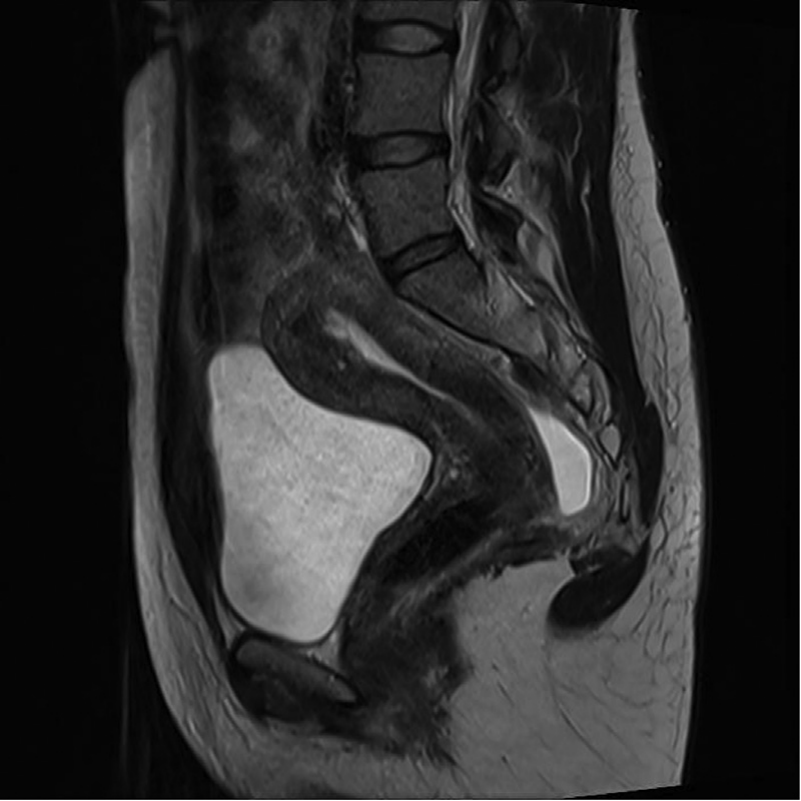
Case 1—Postoperative MRI of the anterior uterine wall showing isodense thickening
